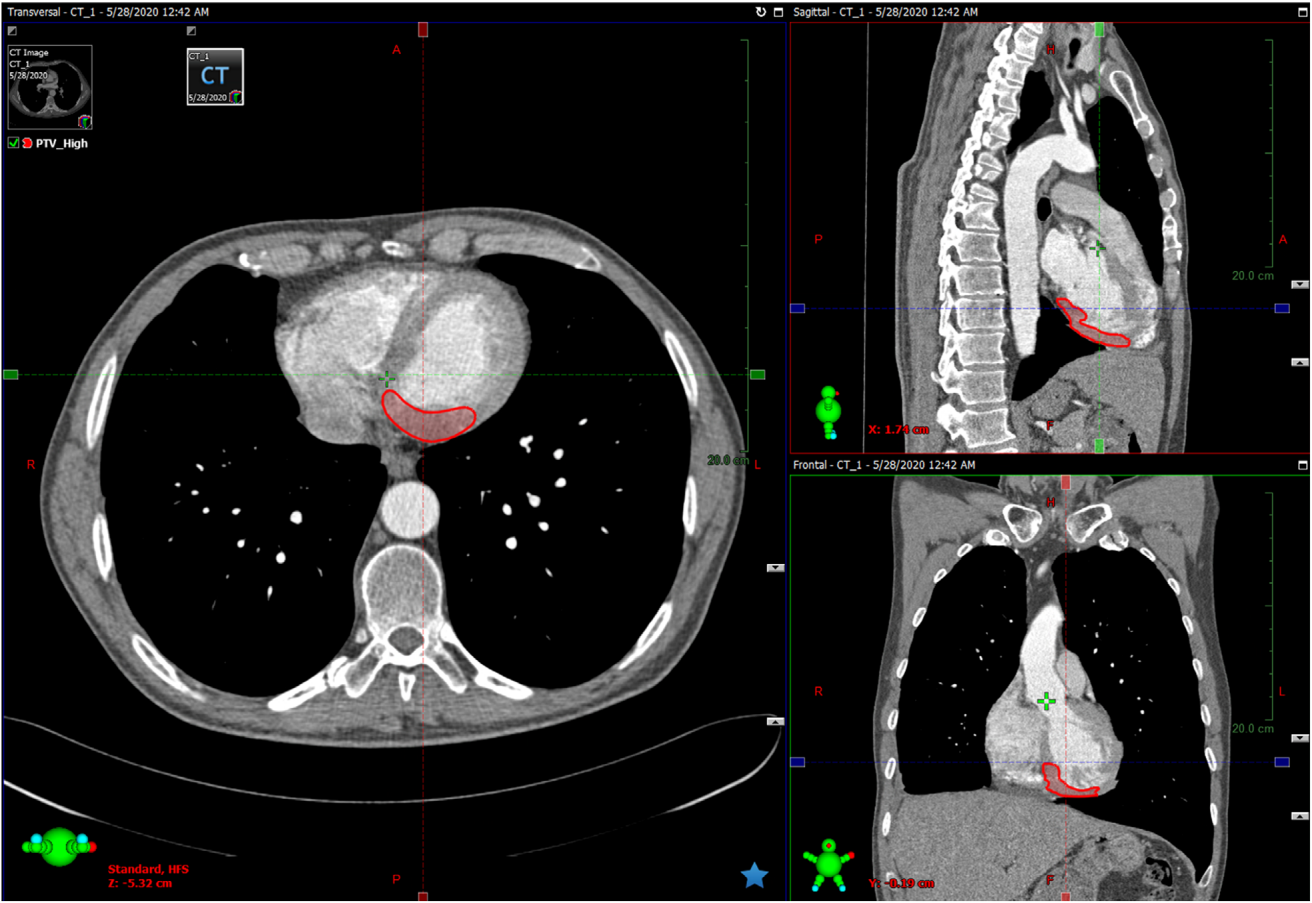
Thorax CT image of one of the patients used in this work for VT‐SBRT planning showing a realistic PTV based on occurrences reported by Neuwirth et al.^19^
